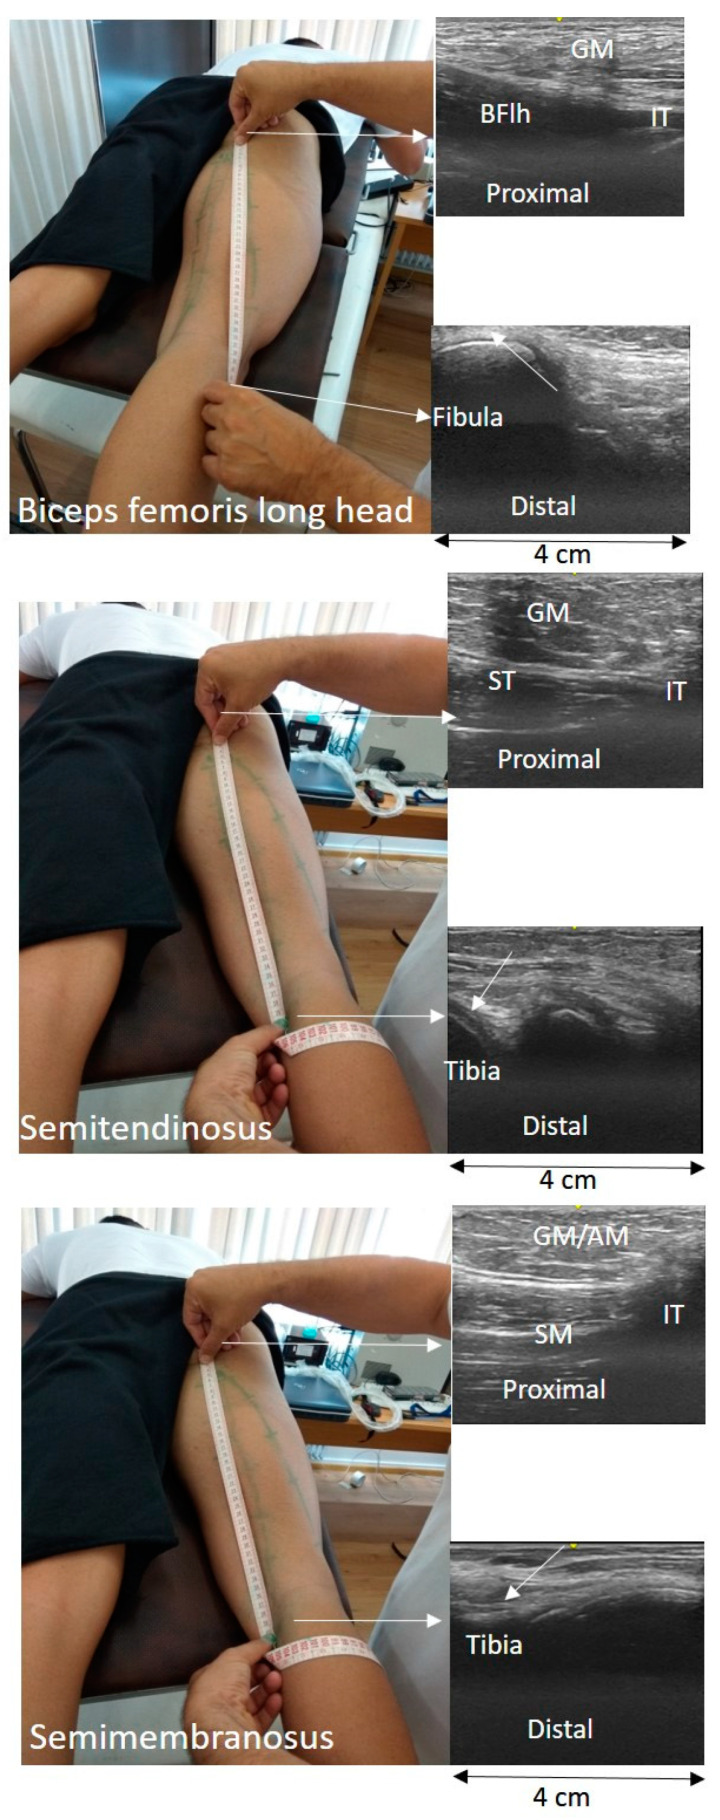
Measurement of muscle-tendon unit length using the tape-ultrasound (TAPE-US) technique. In each testing position, the distal origin and proximal ends of the biceps femoris long head (BFlh), semitendinosus (ST), and semimembranosus (SM) were first identified and marked on the skin (arrows indicate tendon origin). The path of the muscle-tendon unit was drawn on the skin surface with the assistance of US images obtained as the probe moved slowly from the proximal to the distal end. Subsequently, the distance between the two ends was measured using a tape measure (GM = gluteus maximus, AM = adductor magnus, and IT = ischial tuberosity).
